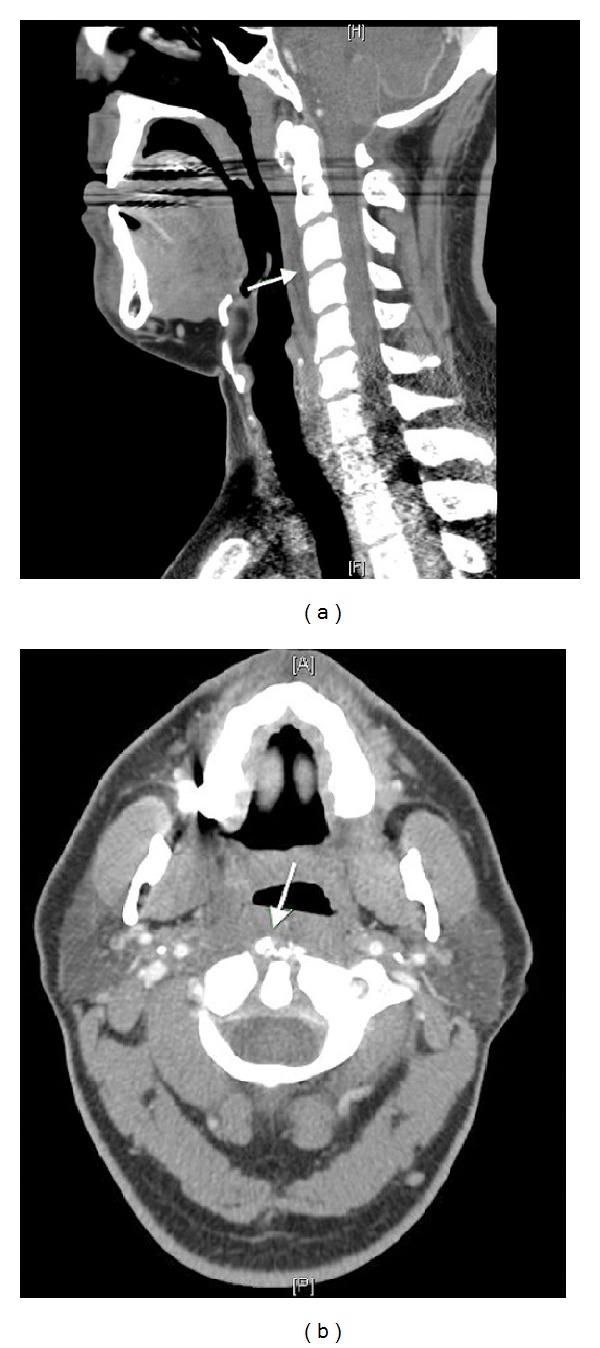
(a) Sagittal CT neck with IV contrast shows a prevertebral fluid collection (arrows). (b) Axial CT neck with calcifications of the longus coli tendon (arrow).
